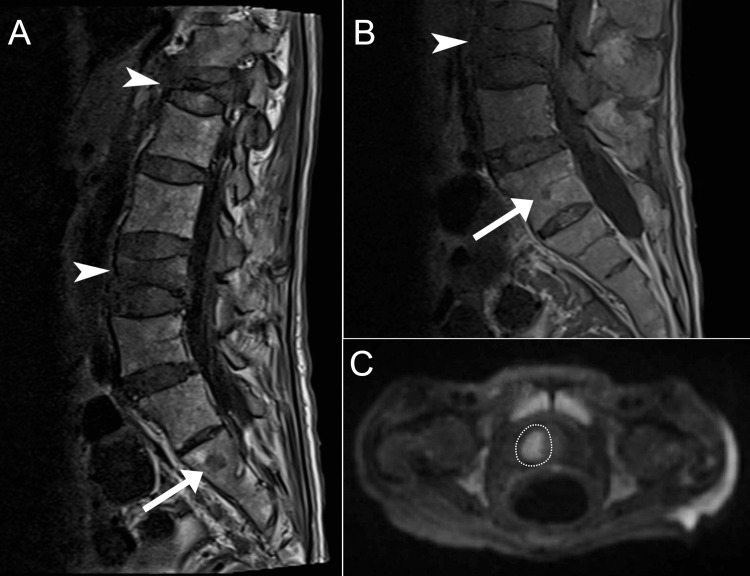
Magnetic resonance imaging of case 2. Lumbar spine MRI T1-weighted images (sagittal, A; parasagittal, B) showing compression fractures of T12 and L3 (arrowheads) and hypointense lesions in L5 and S1 (arrows), suggestive of metastasis. The diffusion-weighted axial pelvic MRI (C) shows a 2.2x1.6-cm area of marked diffusion restriction in the right basal anterior transition zone of the prostate (dotted line).
